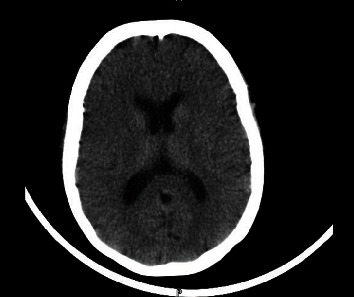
Chest X-ray showing small consolidations in both lower lobes with linear atelectatic changes.
